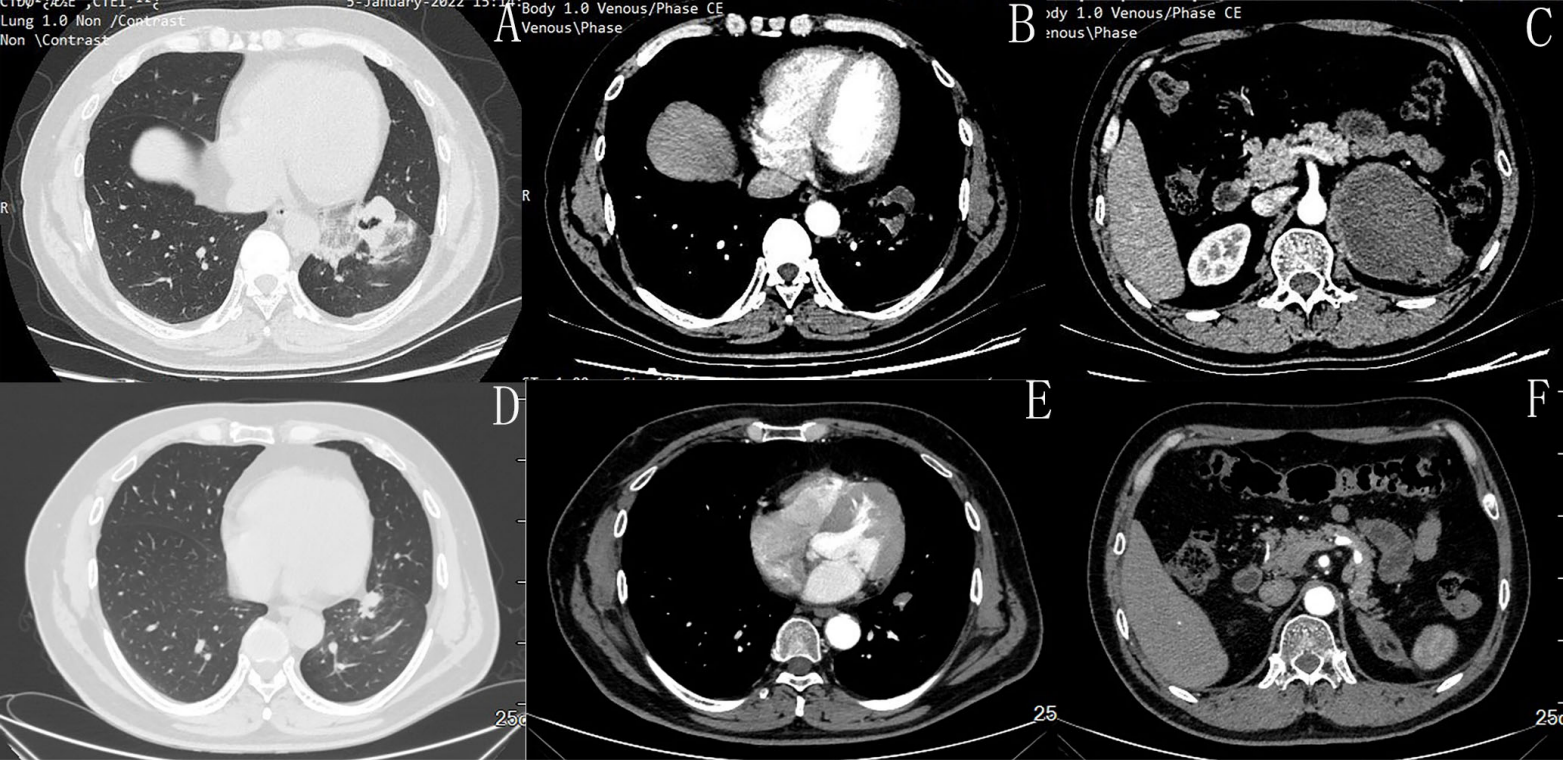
Images before chemotherapy combined with immunotherapy(January 2022). (A) Pulmonary window:primary tumor in the lower lobe of the left lung, measuring 4.9 cm × 3.1 cm. (B) Pulmonary window: left lower lobe lesion. (C) Adrenal metastatic lesion, measuring 9.8 cm × 7.2 cm. (D–F) The most recent CT scan images (September 9, 2025). primary tumor in the lower lobe of the left lung and adrenal metastatic lesion.
